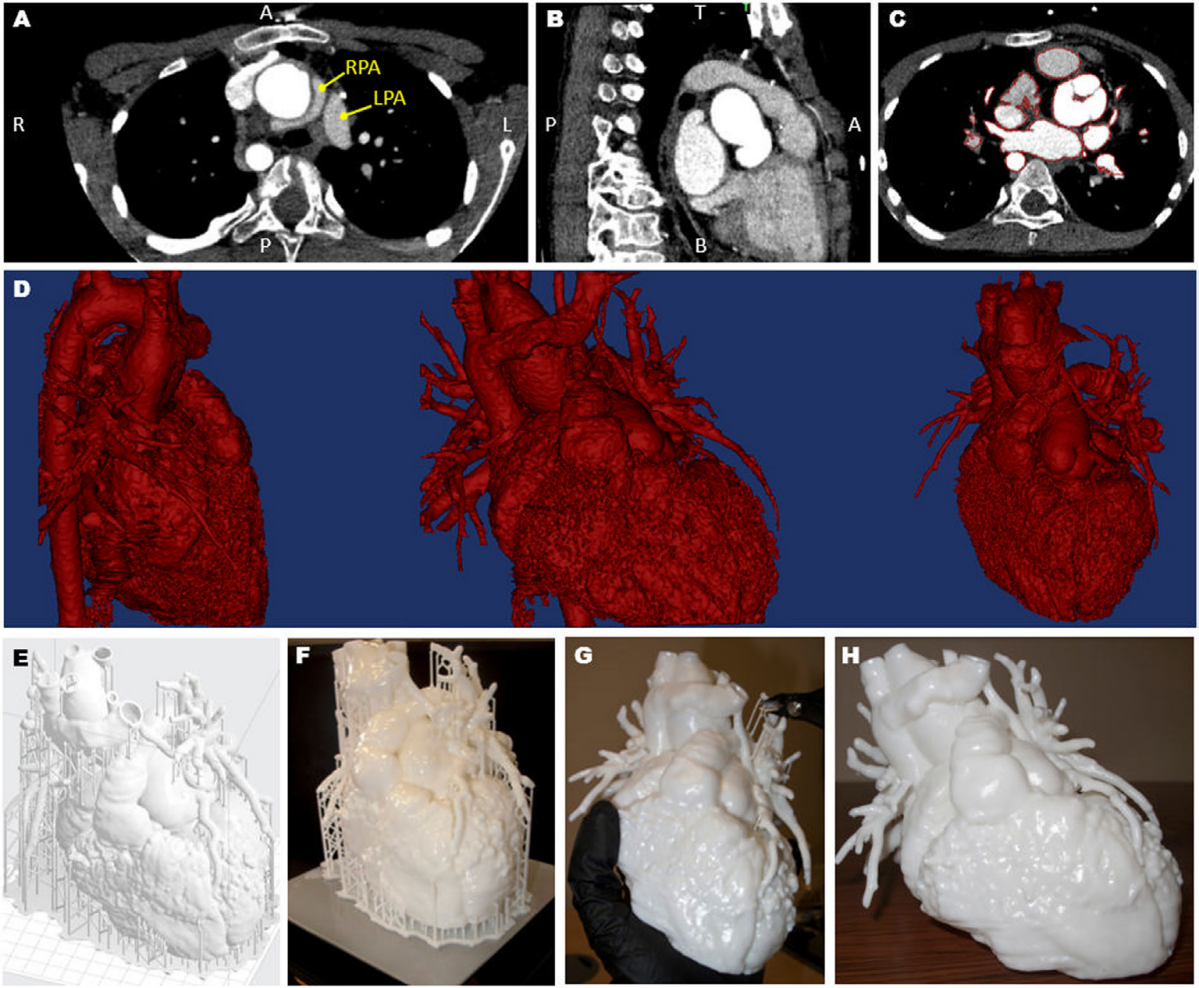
Views of the computed tomography imaging dataset (A–C) including highlight of the position of the left and right pulmonary artery (LPA and RPA), showing the RPA wrapping around the aorta (A); the red outline in panel (C) shows the regions included in the three-dimensional (3D) model; the data are then rendered in 3D [(D), showing different views]; a scaffold (E) is built around the model prior to 3D printing (F) and the removed (G), resulting in the final patient-specific model (H).
